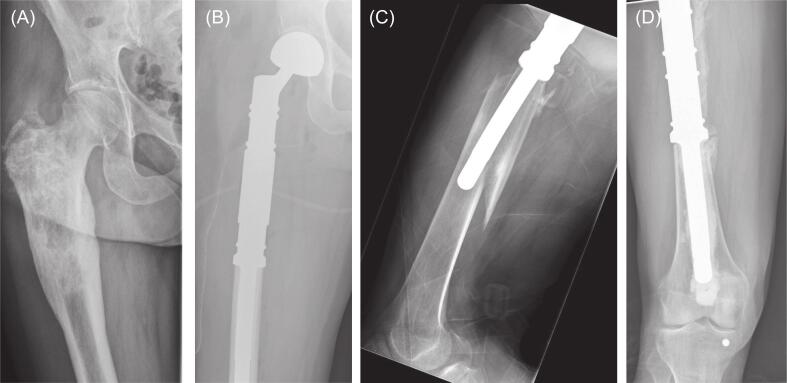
3 A: Preoperative radiograph of a chondrosarcoma of the proximal femur in a 60-year old female patient. 3B: Postoperative radiograph one day after surgery, no complications reported. 3C: Periprosthetic fracture of the femur diaphysis ten years after surgery. 3D: Three months after revision of reconstruction with a cemented stem, resection of the fracture, and extension of MUTARS prosthesis.
